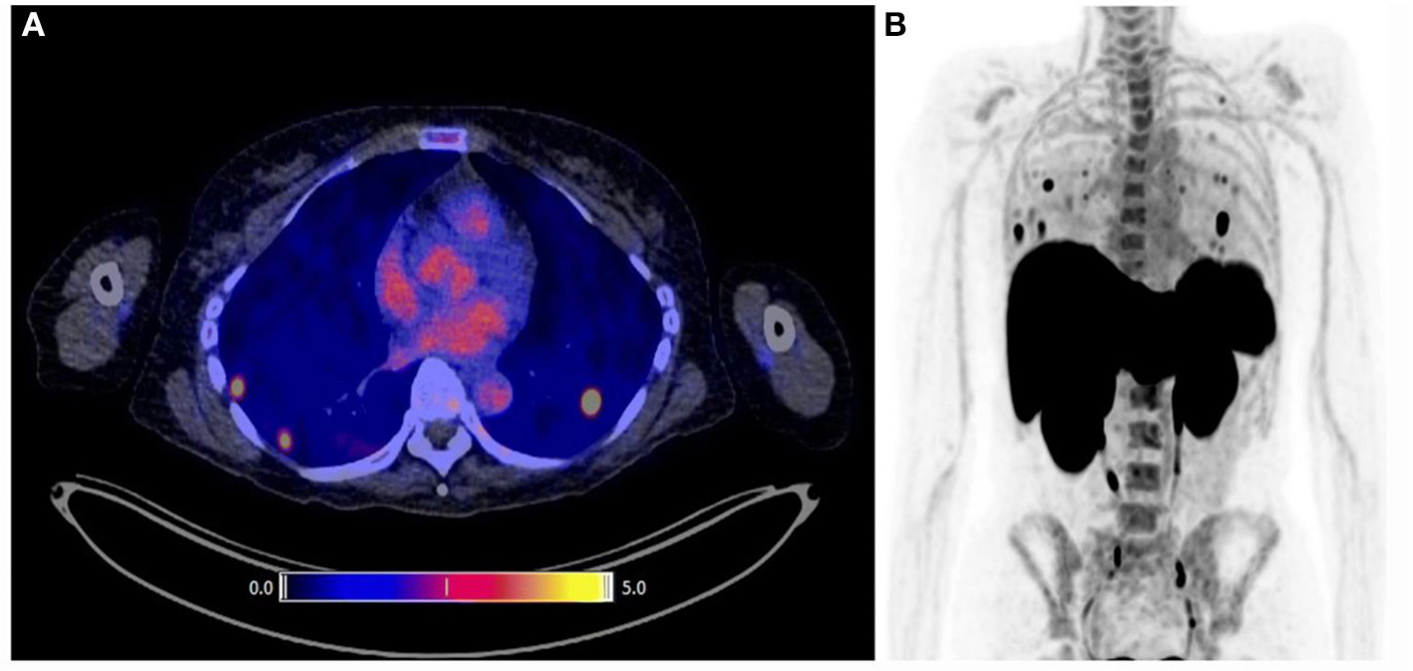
Representative [^18^F]FB-IL2 PET images of human melanoma. (A) Transversal PET/CT image of three regions showing high [^18^F]FB-IL2 uptake. (B) MIP image of the same patient showing multiple areas of high radiotracer accumulation in the lungs. Reproduced from the open access article in ref. (158) under the Creative Commons license (http://creativecommons.org/licenses/by/4.0/).
